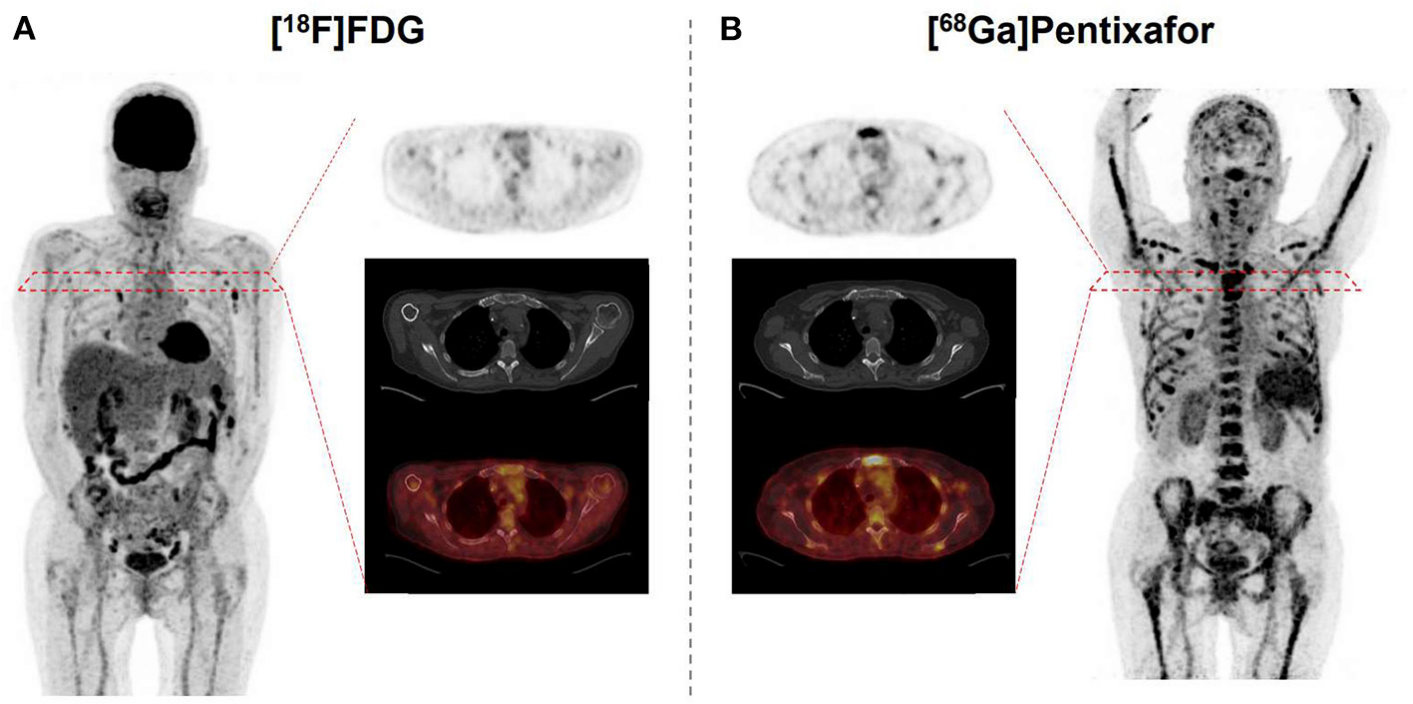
67 year-old patient afflicted with multiple myeloma. Relative to [^18^F]fluorodeoxyglucose (FDG) (A), C-X-C motif chemokine receptor (CXCR4)-targeted [^68^Ga]Pentixafor (B) reveals more sites of disease, as displayed on both the maximum intensity projection and transaxial slices of PET (top) and PET/CT (bottom). Standardized uptake values range from 0 to 5 on both PETs.
